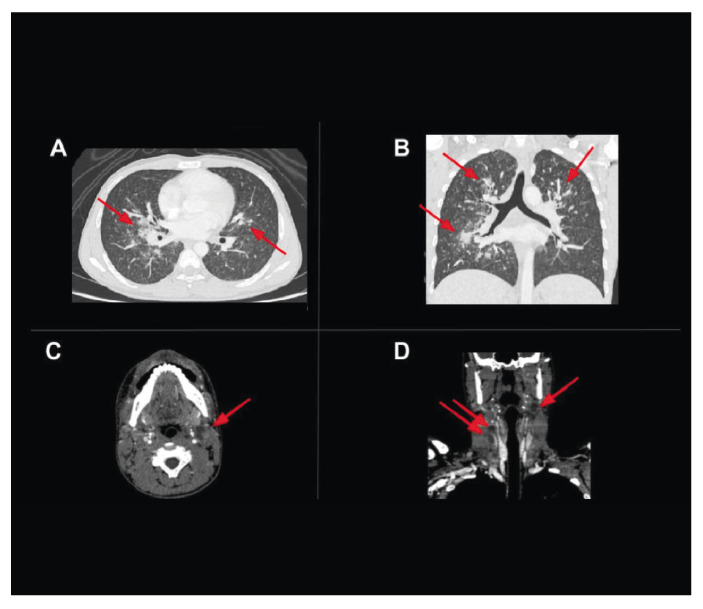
A,B: Axial and coronal computed tomography (CT) of the chest showing lung parenchyma with multiple, widely distributed micronodules with a miliary pattern and areas of peri-hilar consolidation (red arrows). C,D: Axial and coronal CT of the neck showing bilateral lymphadenopathy at multiple cervical levels, and the formation of conglomerates in some regions, with necrotic/liquefied centers (red arrows).
